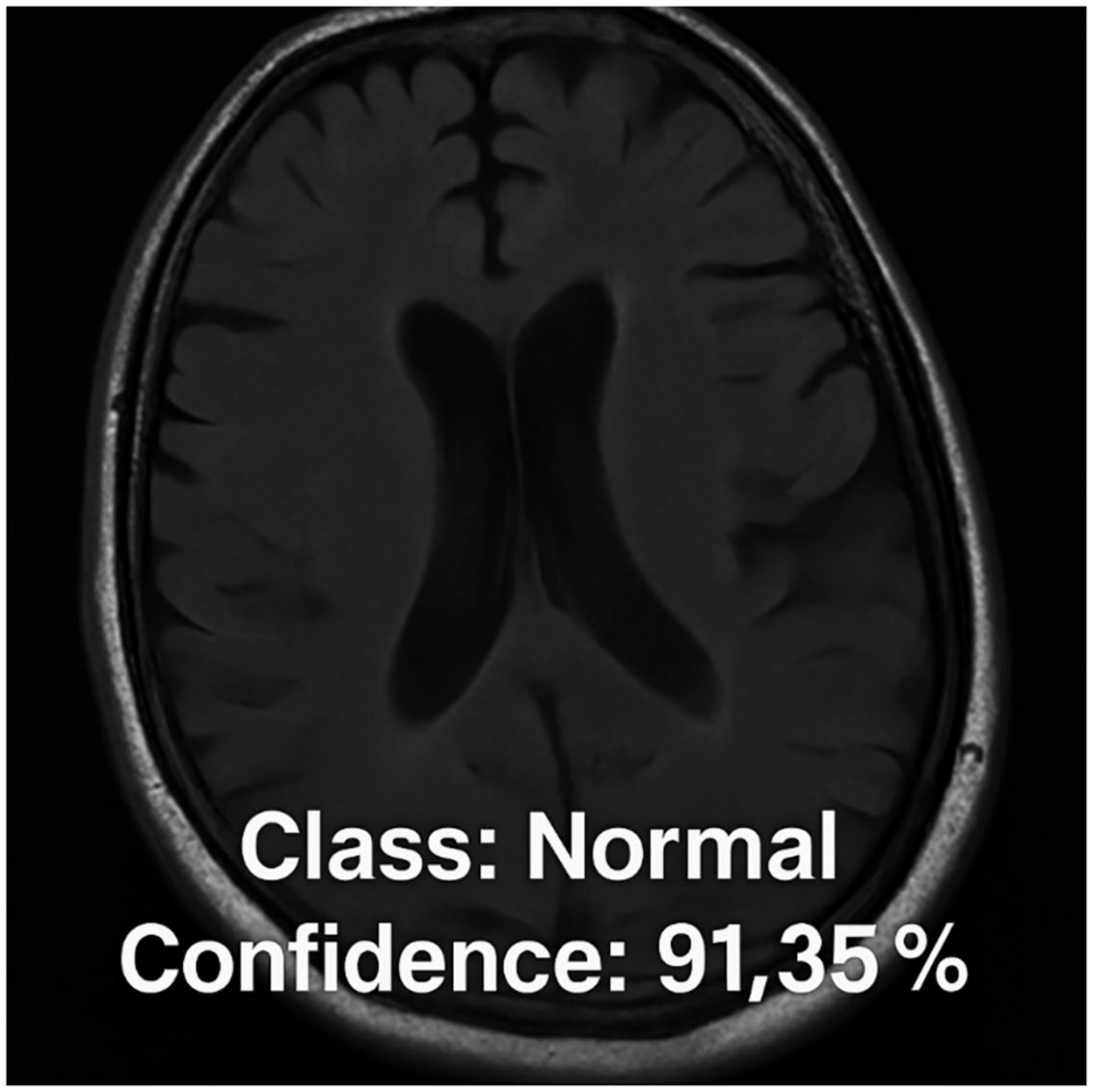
Example of the FastAPI server response: the classification output from the CNN model (“Normal” with 91.35% confidence) is overlaid on the input MRI brain scan and returned as the final response image.
